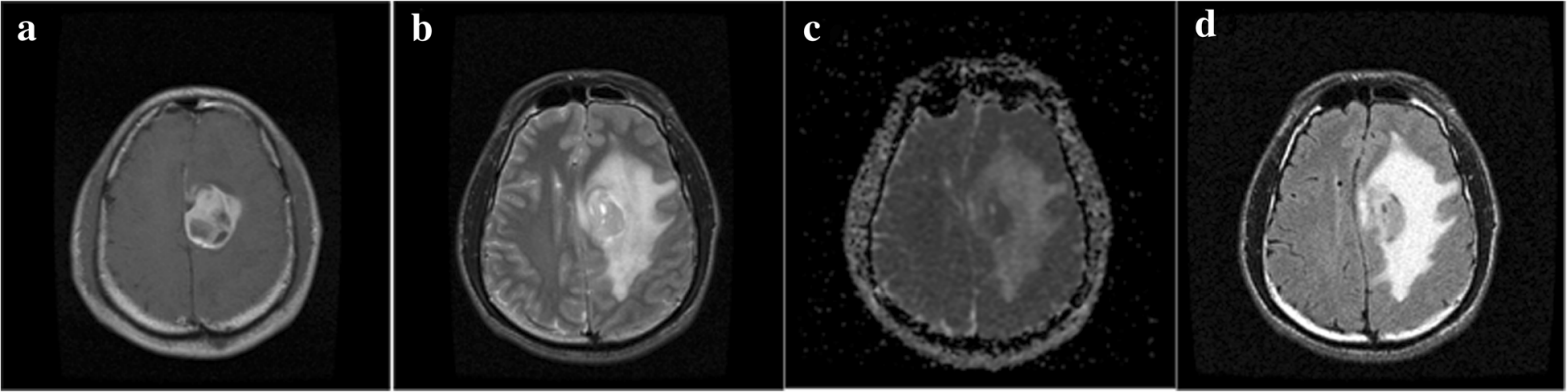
Multimodal images of a patient with astrocytoma. (a) T1-weighted contrast-enhanced image, (b) T2-weighted image, (c) apparent diffusion coefficient (ADC) image, (d) fluid attenuated inversion recovery (FLAIR) image
